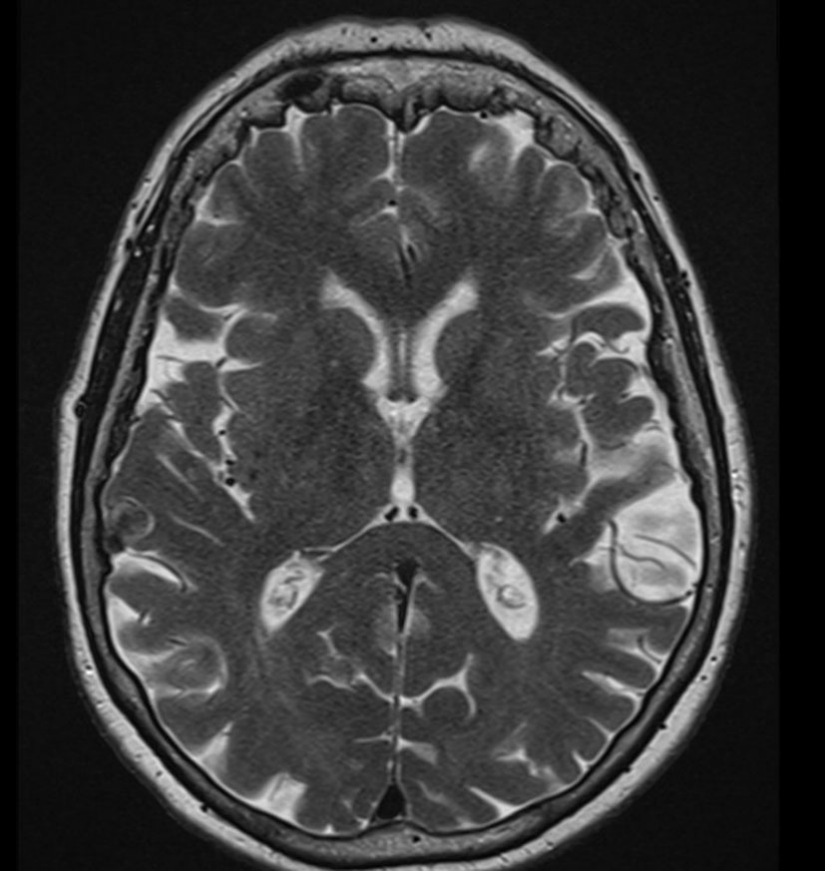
Magnetic resonance imaging. Magnetic resonance imaging (MRI) of the brain shows minor cerebral microangiopathy with periventricular vascular lesions in the white matter.
